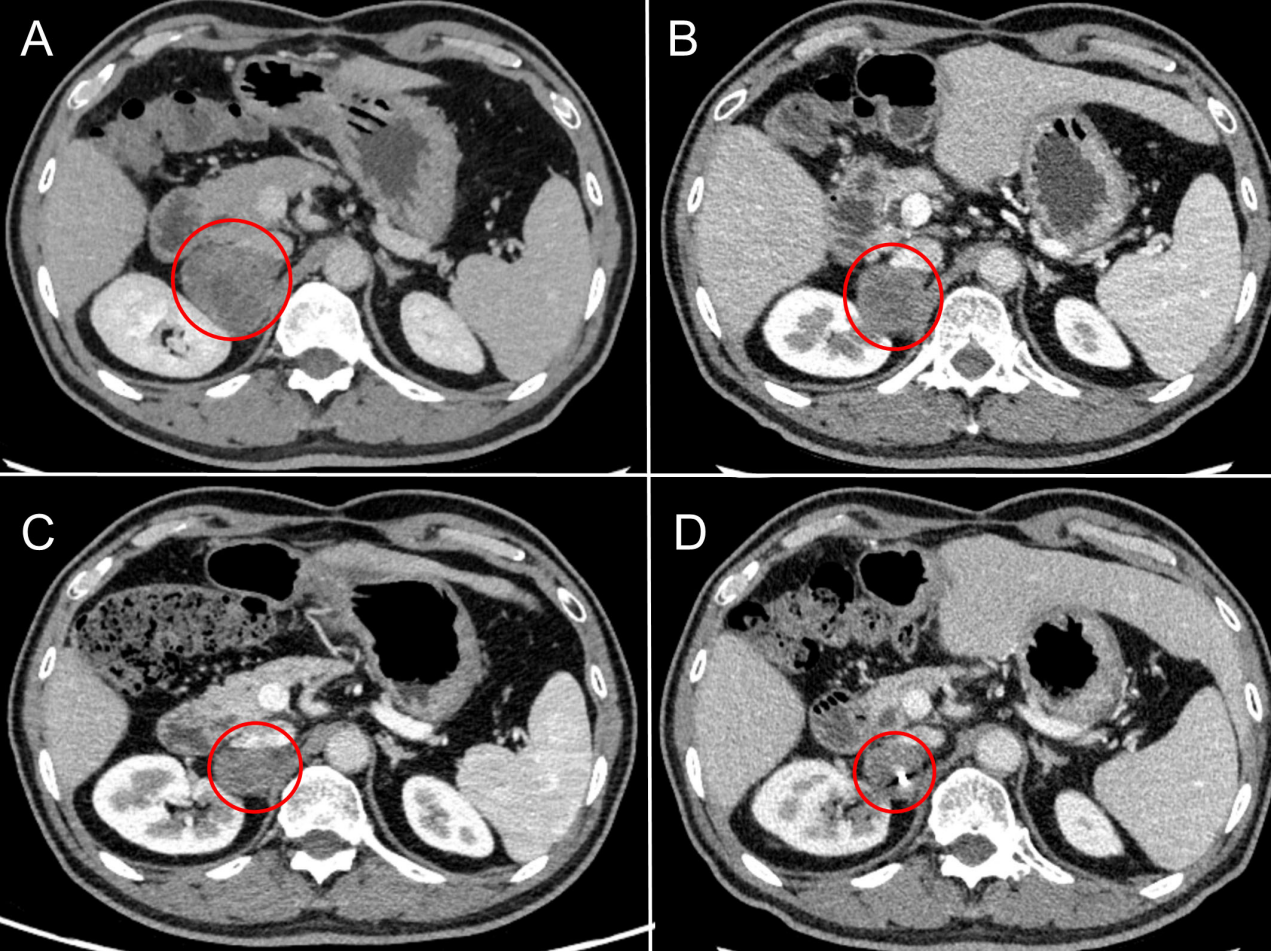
Radiographic changes in adrenal metastases under sequential local interventions. (A) Pre-treatment baseline (March 2020): CT scan revealing a new right adrenal metastasis. (B) After cryoablation (February 2021): CT image post-cryoablation shows a reduction in lesion size and density, indicating successful local tumor control. (C) After CyberKnife radiosurgery (February 2022): CT image demonstrates well-defined radiation-induced changes surrounding the lesion. (D) After odine-125 seed implantation (March 2023): CT image confirming the precise intra-tumoral placement of radioactive seeds.
